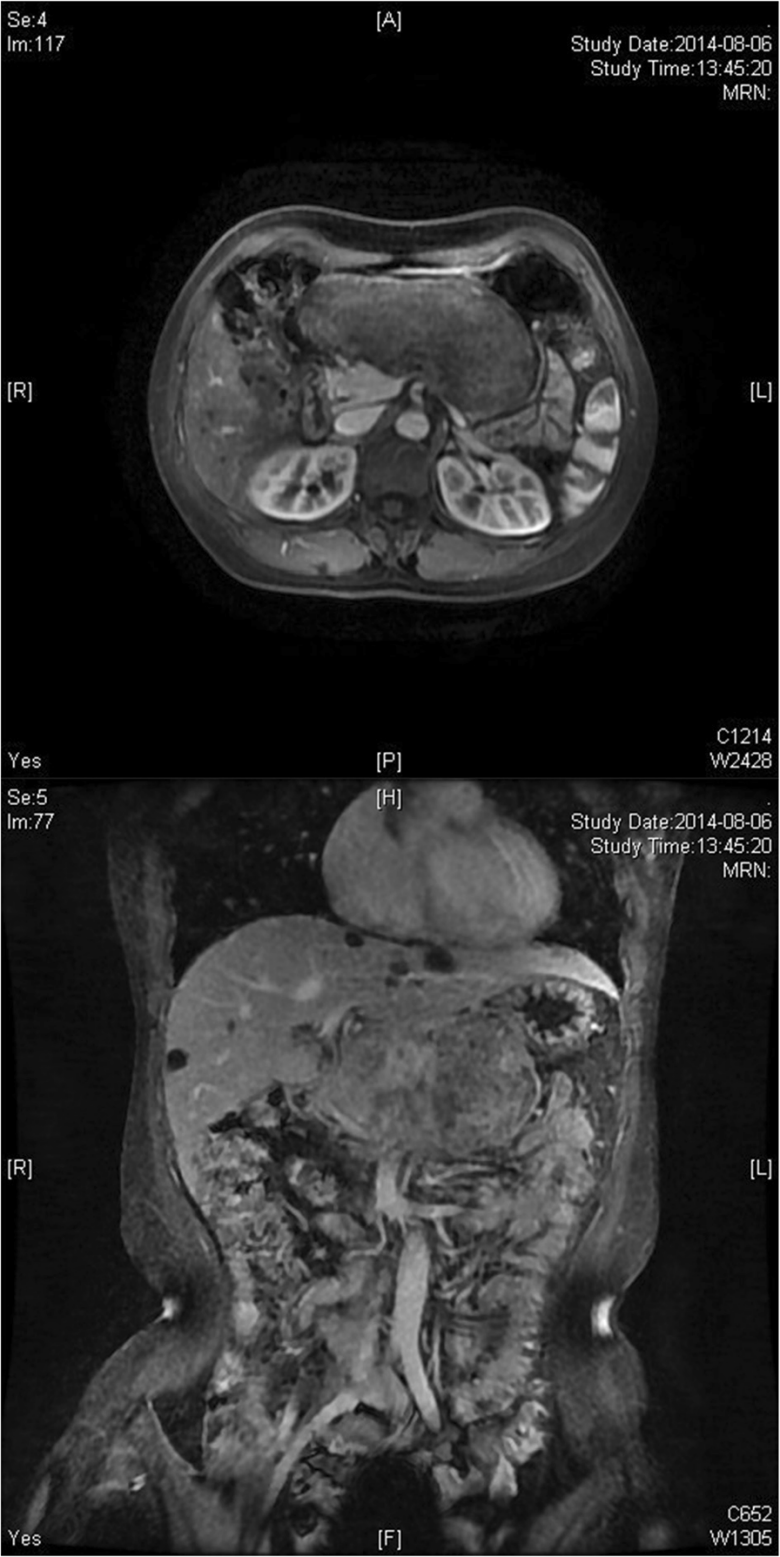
Abdominal dynamic enhanced MRI showing a long T1 signal shadow lesion in the body of the pancreas
